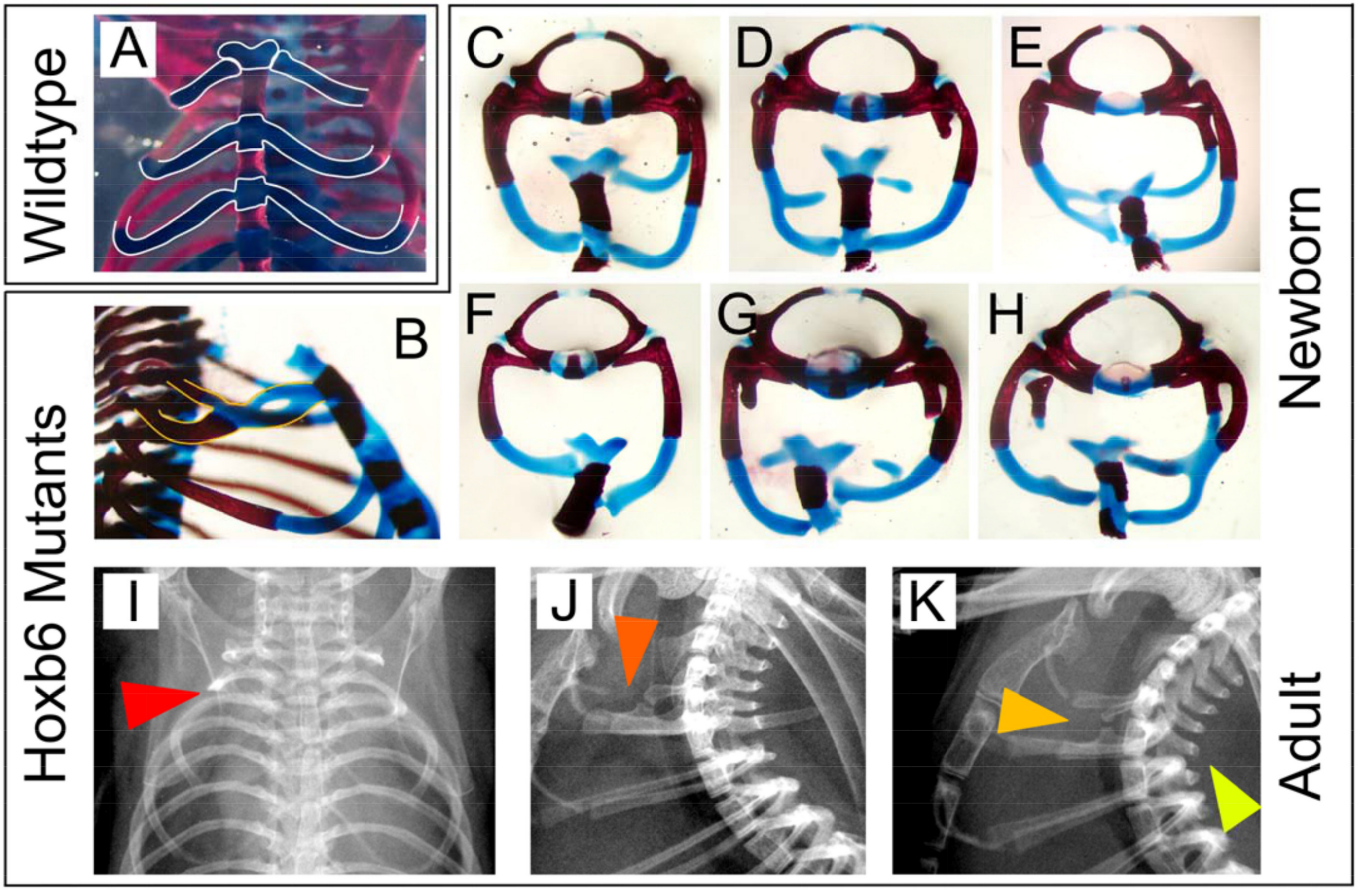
Features of rib development and sternal articulation in Hoxb6^hd^ mutants. Panel A: Newborn wildtype skeleton with outlines (white) of cartilaginous portions of the ribs and sternum. Panels B-K: Hoxb6^hd^ mutant skeletons from newborns (B-H) or adults (I-K). Panel B: Note the crossover of rib from the eighth vertebra and fusion with the rib from ninth vertebra, bifurcation of cartilage, and aberrant attachment of fused cartilage, and fusion of the first two sternebrae. Panels C-H: Different combinations in individuals of absent or rudimentary ribs, unilaterally (C-E) or bilaterally (F-H), defective formation of rib cartilage (D, E, G, H), crossovers (D, E, H), fusions (E, H), bifurcations (D, E, H), aberrant articulation to the sternum (C-H) and off-set sternal attachment of the ribs (H). All preparations contain the eighth and ninth vertebrae, except in Panel F, which represents the ninth vertebra. Panels I-K: X-rays of individual adult Hoxb6^hd^ mutants. Panel I: frontal view; note truncation of first rib unilaterally (red arrow). Panel J: lateral view, orange arrow points to crossover and defective sternal rib cartilage. Panel K: lateral view, dark yellow arrow points to crossover and unilateral absence of first rib, bright yellow arrow points to absence of spinous process on the ninth vertebra.
